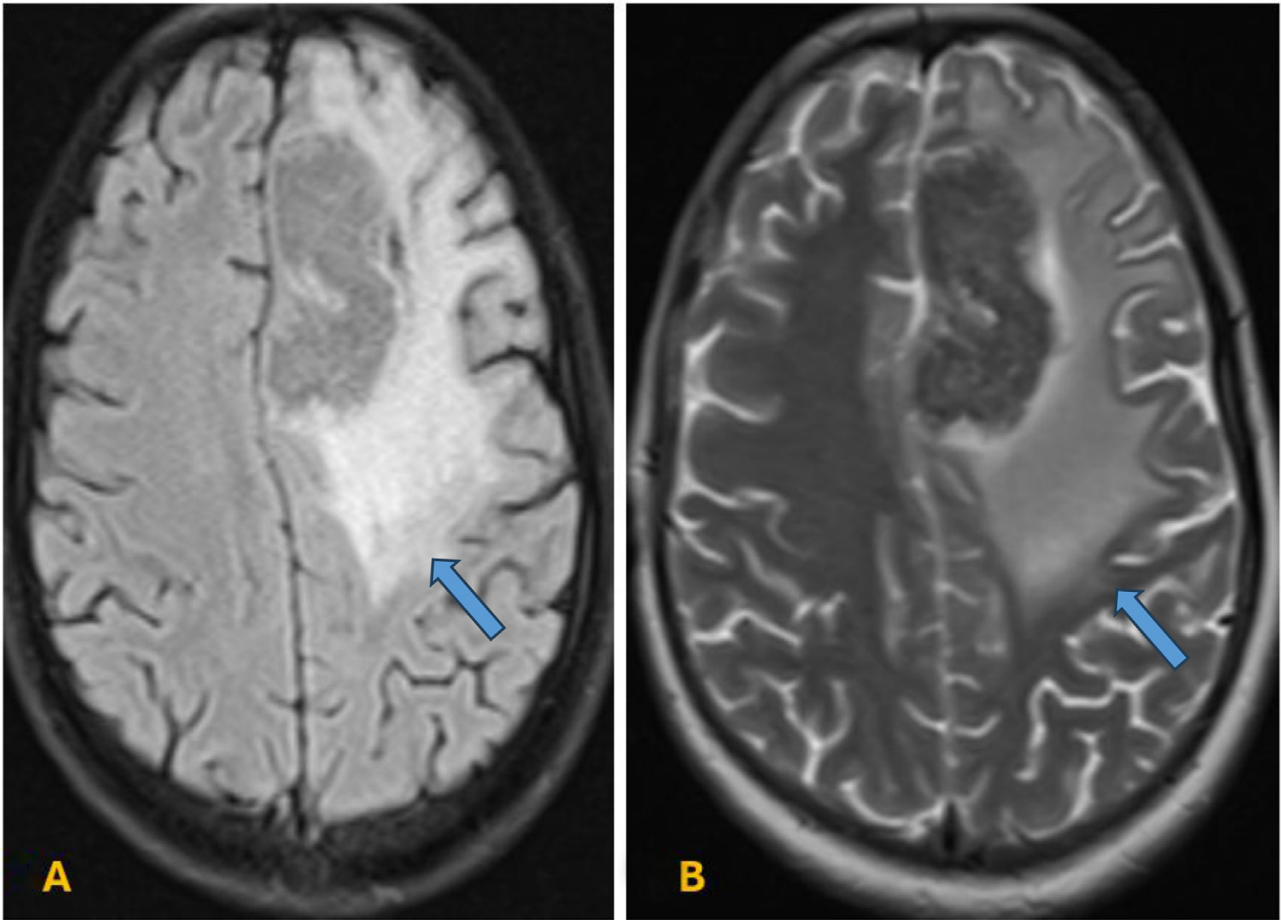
(A–B) Axial T2-weighted and FLAIR images highlighting extensive perilesional edema surrounding the lesion (blue arrow).
